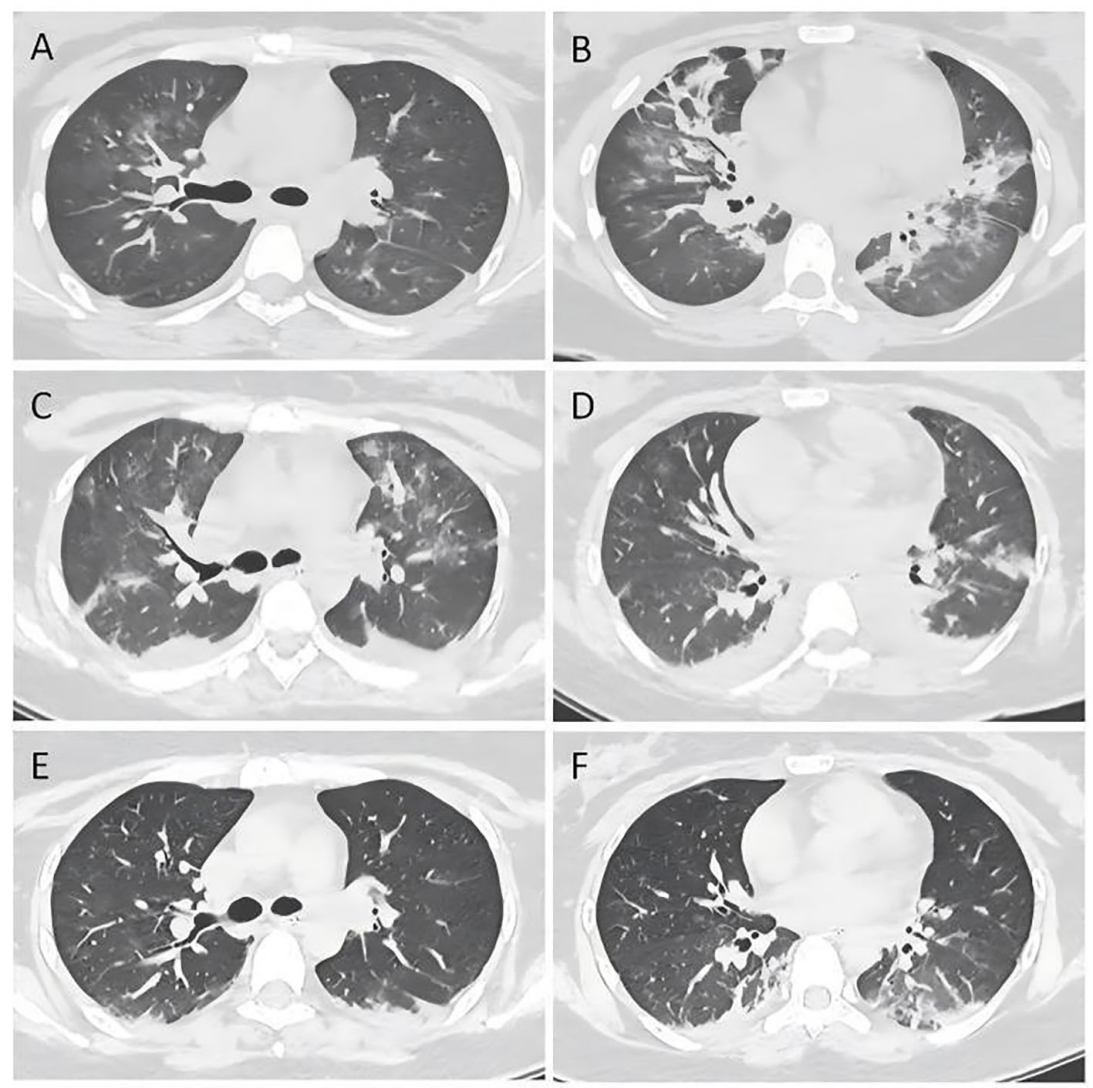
Chest computed tomography scan on day 2 (A, B), day 6 (C, D) and day 12 (E, F).
